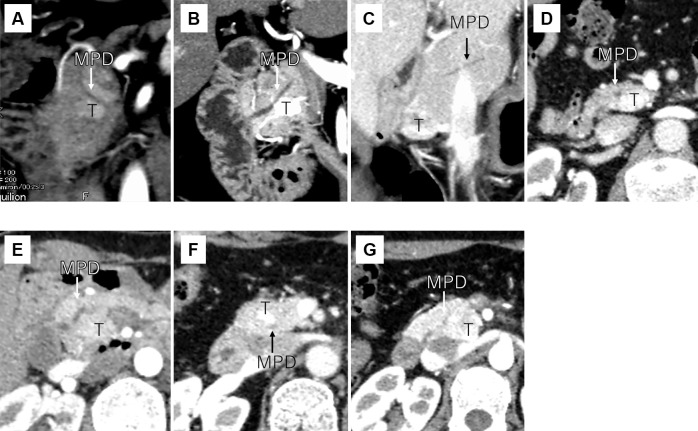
Relationship between tumor location and MPD on enhanced computed tomography (A) Patient 1. (B) Patient 2. (C) Patient 3. (D) Patient 4. (E) Patient 5. (F) Patient 6. (G) Patient 7. T: tumor, MPD: main pancreatic duct
